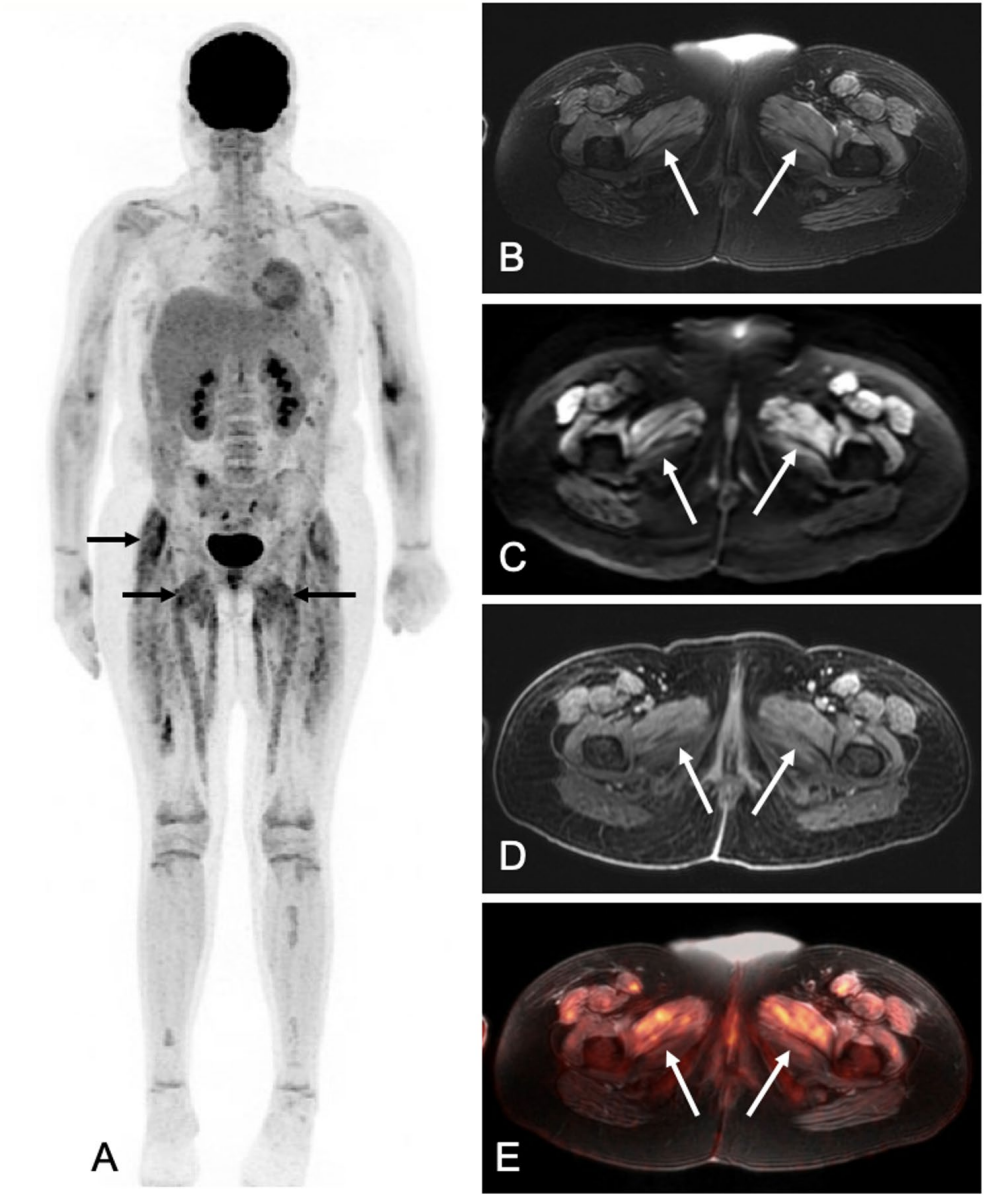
CRS-induced myositis in a 13-year-old girl with relapsed acute lymphoblastic leukemia, noted on 18F-FDG PET/MR at day 28 after CAR T-cell infusion. A) 18F-FDG PET MIP demonstrates marked 18F-FDG radiotracer uptake in skeletal muscle throughout the body, including the bilateral legs (arrows), consistent with myositis. ​B) Axial T2-weighted MRI at the level of the pelvis shows bilateral diffuse T2-hyperintense edema of muscles of the anterior thigh, including the adductor muscles.​ C) Axial diffusion-weighted MRI at the level of the pelvis shows mildly restricted diffusion in the affected muscles with ADCmean of 980x 10^−6^ mm2/s. D) Axial contrast-enhanced T1-weighted MRI shows mild contrast enhancement of affected muscles. E) Integrated 18F-FDG PET/MR demonstrates contrast enhancement and 18F-FDG radiotracer uptake in the affected muscles, consistent with myositis
